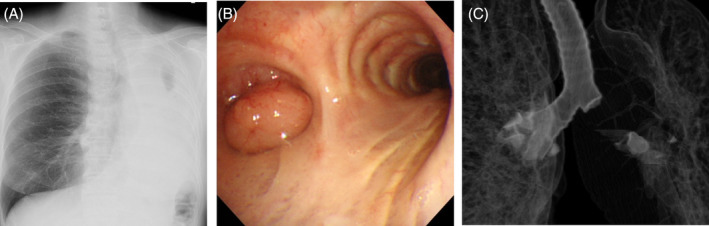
(A) Chest x‐ray demonstrated complete atelectasis of the left lung and mediastinal deviation to the left. (B) Flexible bronchoscopy showed complete obstruction of the left main bronchus by a polypoid tumour. (C) Chest computed tomography revealed a polypoid tumour, originating from left main bronchus and occupying the orifice of the left main bronchus.
